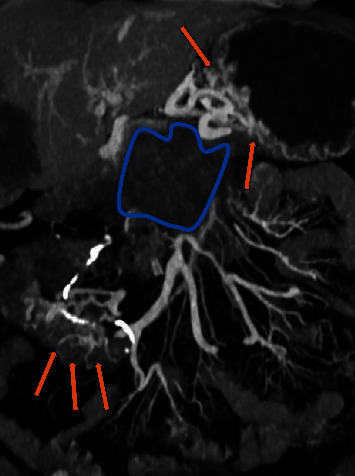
Computerized tomography (CT scan) portal vein phase, coronal view: ill-defined malignant tissue infiltrating the superior mesenteric vein and portal vein (blue outline). Dilated small veins compatible with collateral circulation (ectopic varices) in the terminal ileum (yellow arrows). Tortuous dilated veins are observed at the fundus of the stomach (orthotopic varices) (orange arrows).
